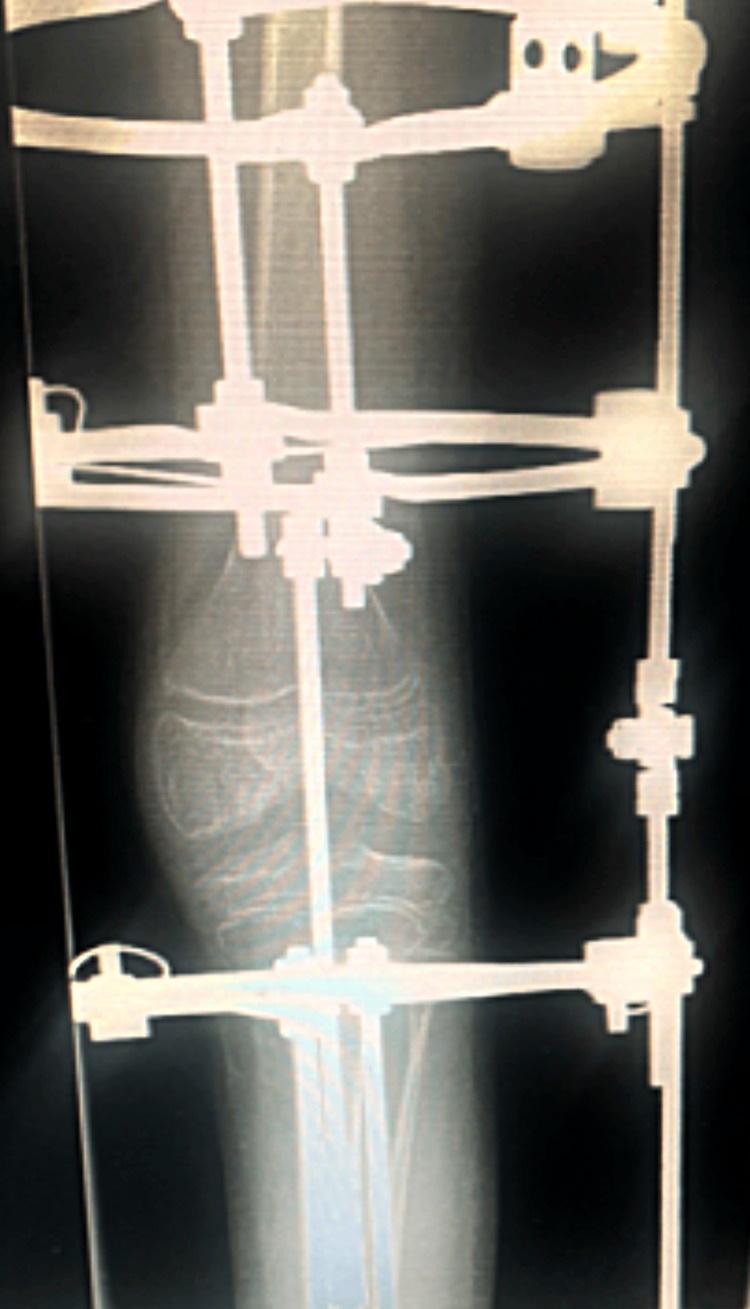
Postoperative anterior X-ray six weeks post-correction of the fixed flexion deformity of the left knee
